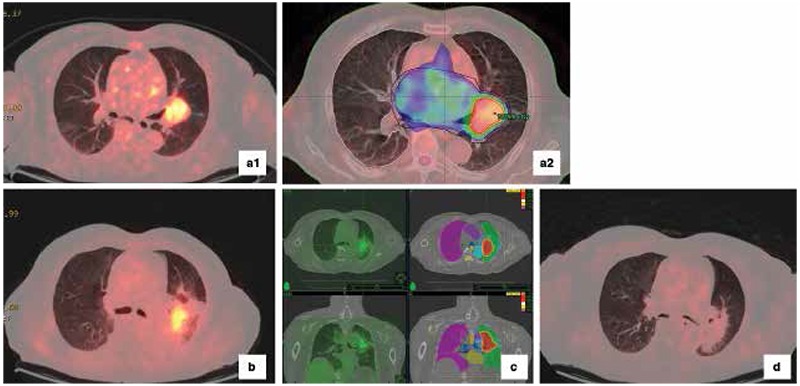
Non-small cell lung cancer, 82 y.o., M patient, a1) Abnormal increased metabolic activity was observed in the mass identified in the left parahilar region and, a2) Intensity modulated radiation therapy planning images by these views, b) Increased metabolic activity compatible with residual mass was seen in positron emission tomography/computed tomography that was applied for treatment-control after four months, c) Plan images belonged to stereotactic body radiation therapy that was applied to the residual mass, d) Pathologic activity was not observed in positron emission tomography/computed tomography after one year (complete metabolic response)
